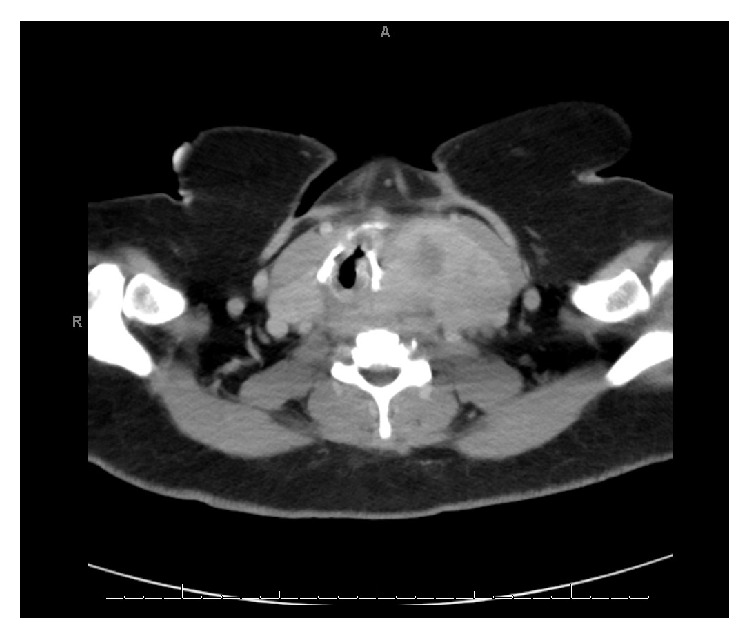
An axial image of the goiter with computed tomography.
